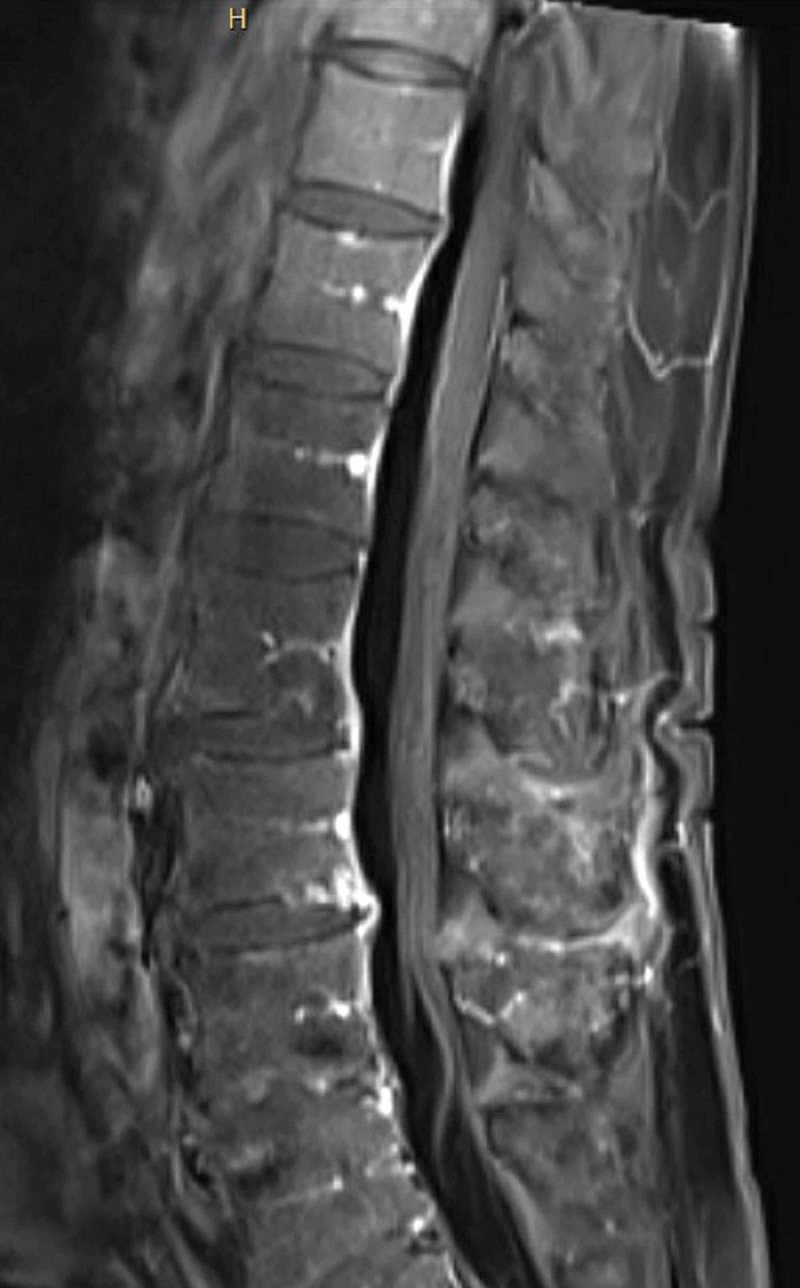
MRI lumbar spine with contrast Sagittal T1 post-contrast MRI lumbar spine demonstrating enhancement of cauda equina nerve roots indicative of Guillain-Barre Syndrome
